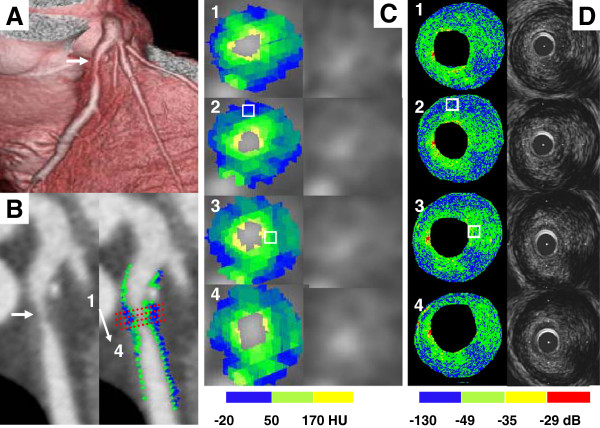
(A) A volume-rendering image of the left anterior descending artery (arrow). A plaque with intermediate stenosis. (B) (Left) Curved multiplaner reconstruction image shows intermediate stenosis in the proximal portion of left anterior descending artery (arrow). (Right) Color-coded curved multiplaner reconstruction image of coronary plaque. (C) (Left) Color-coded cross-sectional multiplaner reconstruction image. (Right) Gray-scale multiplaner reconstruction images. Numbers that identify the images correspond to the cross-section in (B). Regions of interests (white square, 0.5 x 0.5 mm) with homogenous tissue components were selected on IB-IVUS images, and were used to set the corresponding region of interests on cross-sectional multiplaner reconstruction images of multidetector computed tomography. (D) (Left) Integrated backscatter intravascular ultrasound color-coded maps. (Right) Gray-scale intravascular ultrasound images. Numbers that identify the images correspond to the cross-section in (B).
